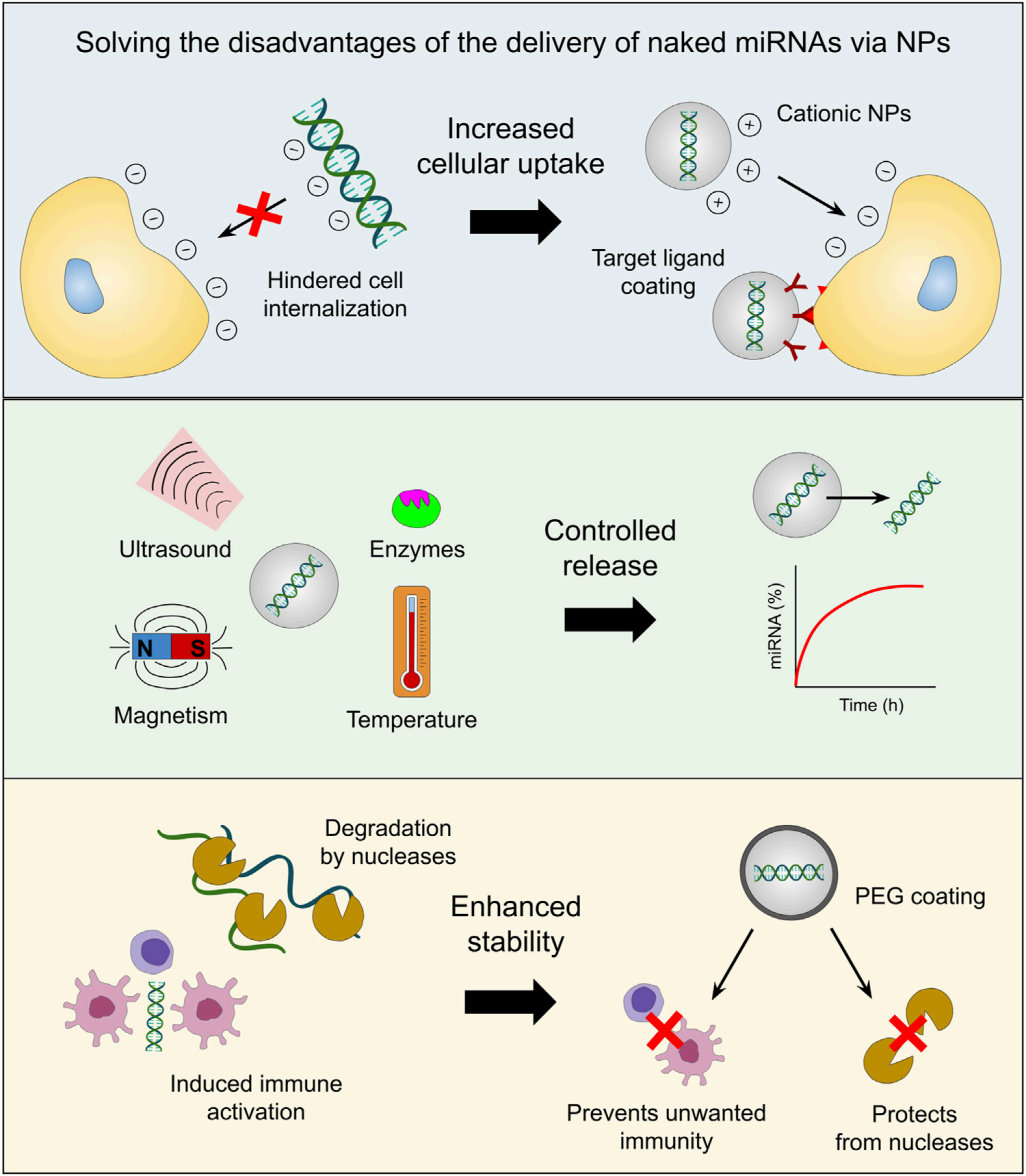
Advantages of the NP-mediated delivery of miRNA-based drugs. Nucleic acids, as well as the cell membrane, are negatively charged, which makes cell internalization of naked miRNAs a challenge. Cationic NPs envelop miRNAs in a positively-charged shield which improves cellular uptake. Moreover, the addition of target ligands in the outer layer of NPs can enhance selectivity and reduce off-target effects. Furthermore, controlled release of the therapeutic cargo can be achieved when the NPs are in contact with stimuli such as specific enzymes, ultrasound, magnetic fields and temperature, to name a few. Additionally, nanoencapsulation boosts miRNA stability by protecting them from nuclease degradation and inhibiting undesired immune activation through the implementation of PEG-based coating.
